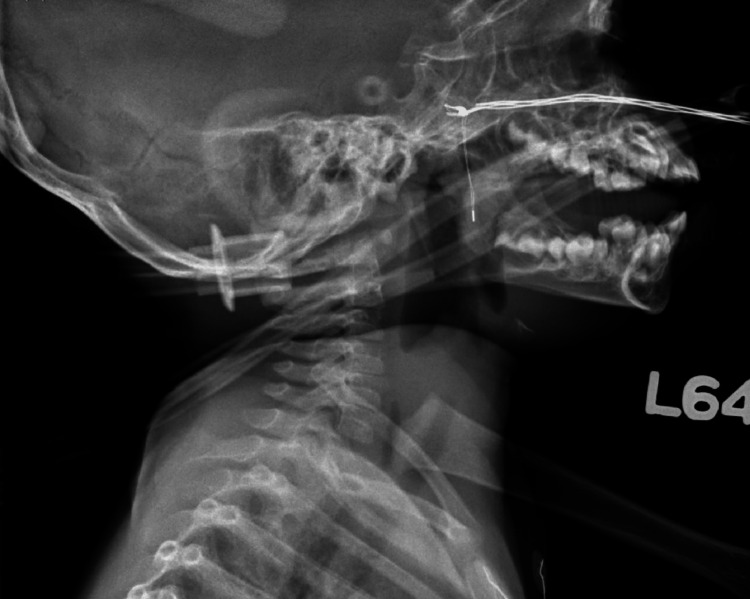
Lateral radiograph showing initial subglottic stenosis and diffuse tracheal narrowing
